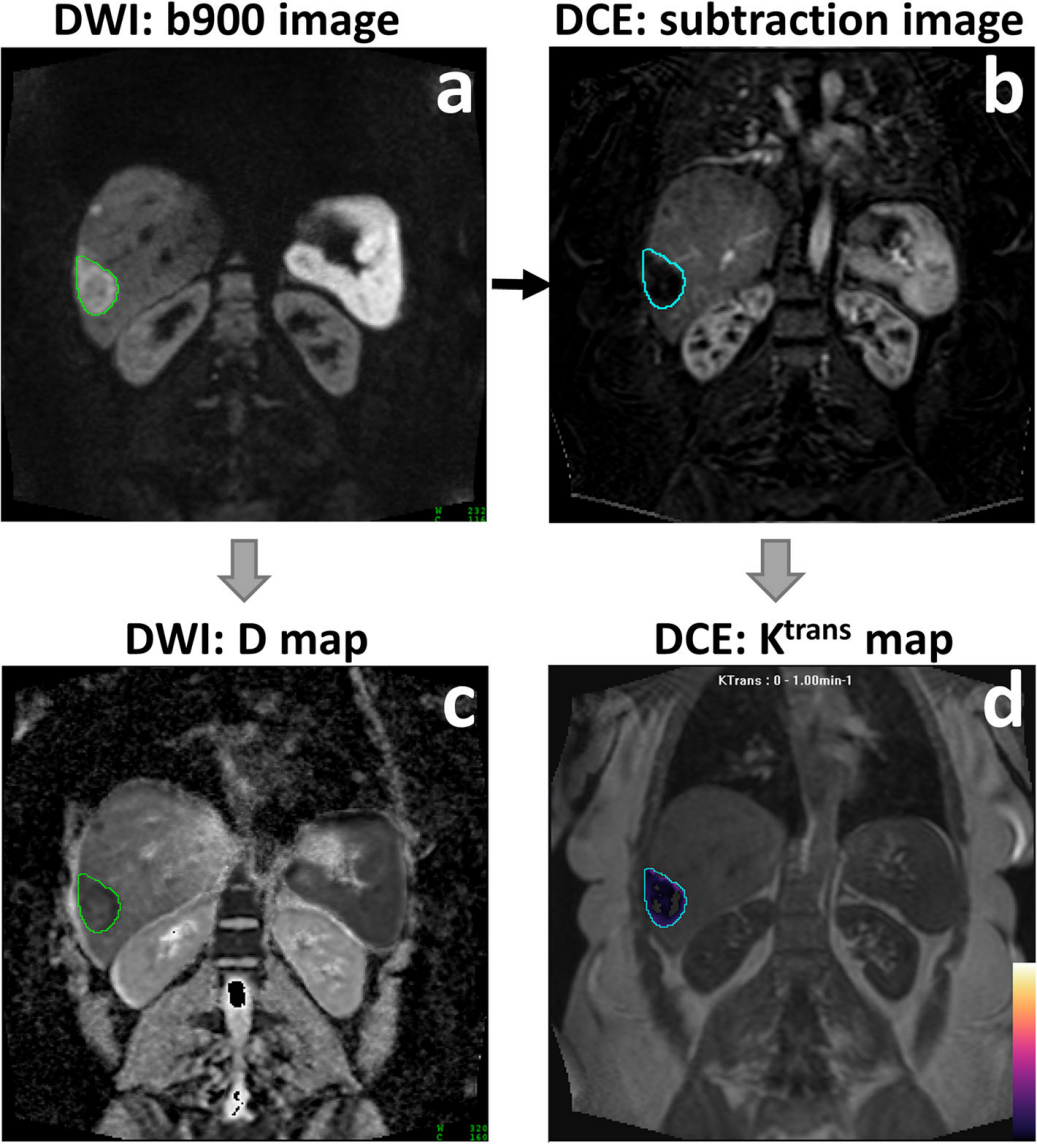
VOI-based data processing (example of one slice out of the 8 evaluated) for a 48 year old female patient with a lesion in segments 7/8 of the liver. VOI was drawn on the highest-b-value image (a), then transferred to the DCE-MRI subtraction image (b). The subtraction image was calculated as the difference between the dynamic image with peak enhancement within the liver parenchyma (dynamic 9/40) and the first pre-contrast image (dynamic 1/40). The VOIs were used in conjunction with each of the computed maps to derive the median values of parameters of interest: D (c) and K^trans^ (d) are shown here. Note that K^trans^ map is shown overlaid on the last dynamic image of the DCE-MRI acquisition (dynamic 40/40)
